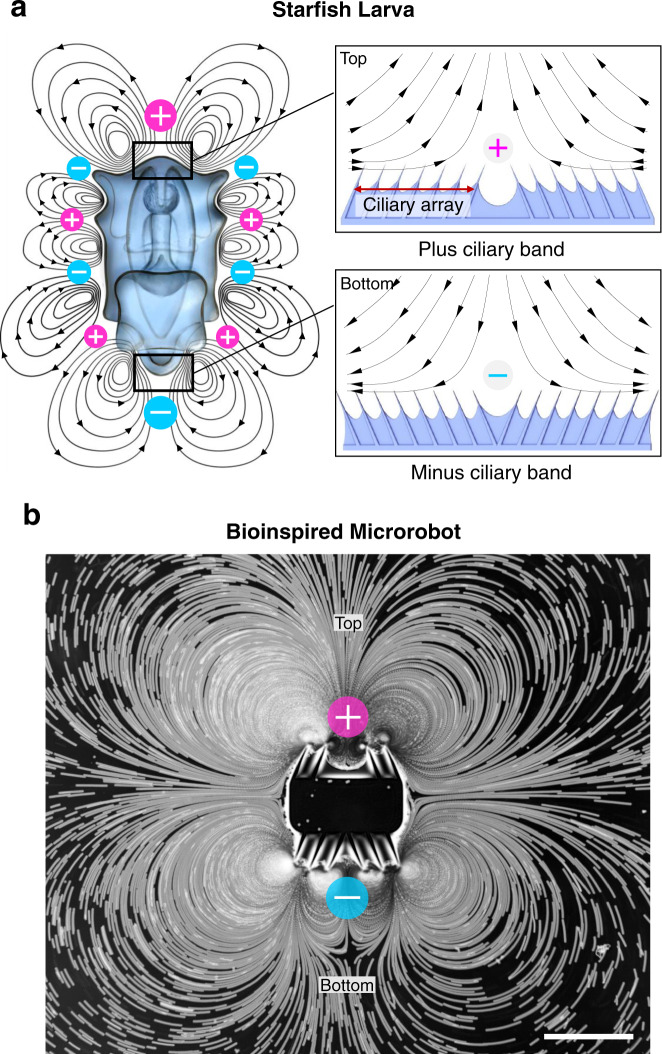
Starfish larva-inspired ultrasound ciliary band designs. a A starfish larva exhibits a complex flow profile of counter-rotating vortices generated by a series of + and − ciliary bands arranged on its body protuberances. Inset: (top) A + ciliary band comprises a pair of angled ciliary arrays that face each other and causes the liquid to flow away on a plane perpendicular to the center of the ciliary band. (Bottom) A − ciliary band consists of two ciliary arrays oriented away from each other and directs the liquid flow to the center of the band. b A starfish-inspired microrobot consisting of a + (top) and a − (bottom) ciliary band placed in a tracer solution. The ciliary bands oscillate when actuated by ultrasound, producing complex flow profiles similar to those observed with its biological counterpart. Scale bar, 250 μm.
